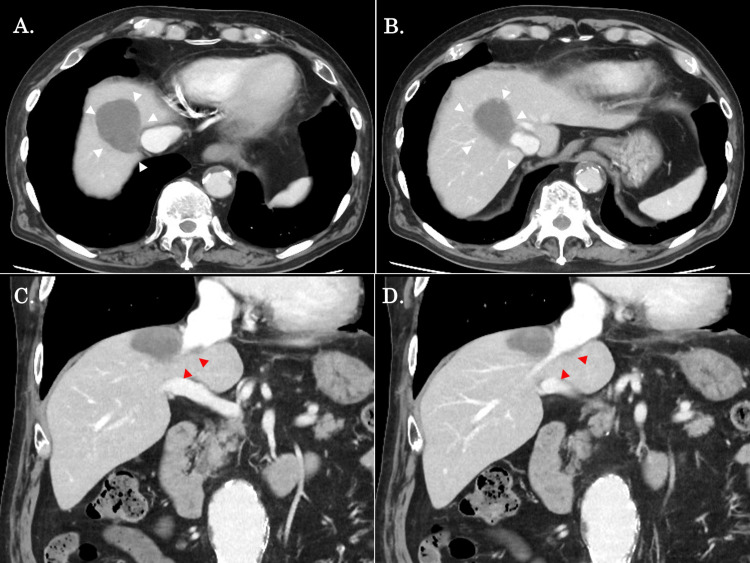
Contrast-enhanced CT findings after the chemotherapy. (A, B) An axial contrast-enhanced CT scan revealed a reduction in tumor size of 48 × 39 mm (white arrows). (C, D) Coronal sections showed that the tumor was still in contact with the right hepatic vein, but the proximity to the inferior vena cava and middle hepatic veins was alleviated (red arrows).
